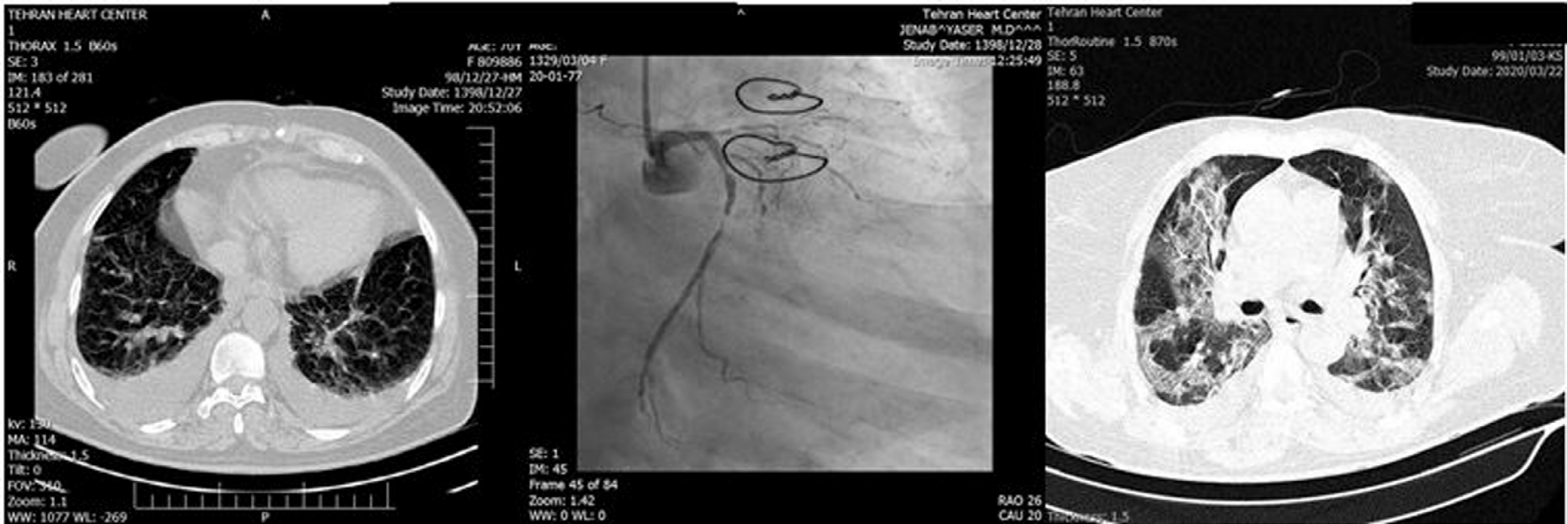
Sorted by time. Left panel: First on‐admission chest computed tomography scan showing bilateral pleural effusion. Middle panel: 10 h after admission, coronary angiogram, post‐CABG, left main is cut, and very significant stenosis in left circumflex artery. Right Panel: Second chest computed tomography scan 3 d later; ground‐glass and patchy areas in favor of COVID‐19 infection
